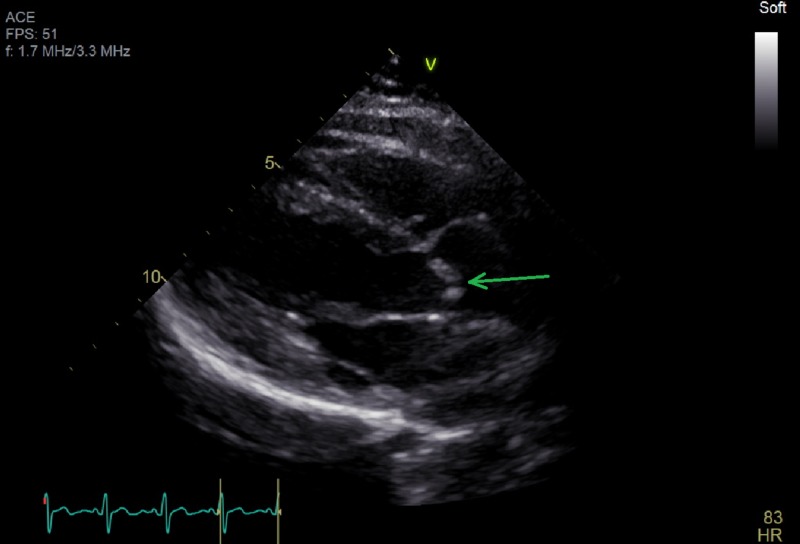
Parasternal Long Axis View Showing Eccentric Closure of Aortic Valve (arrow)
